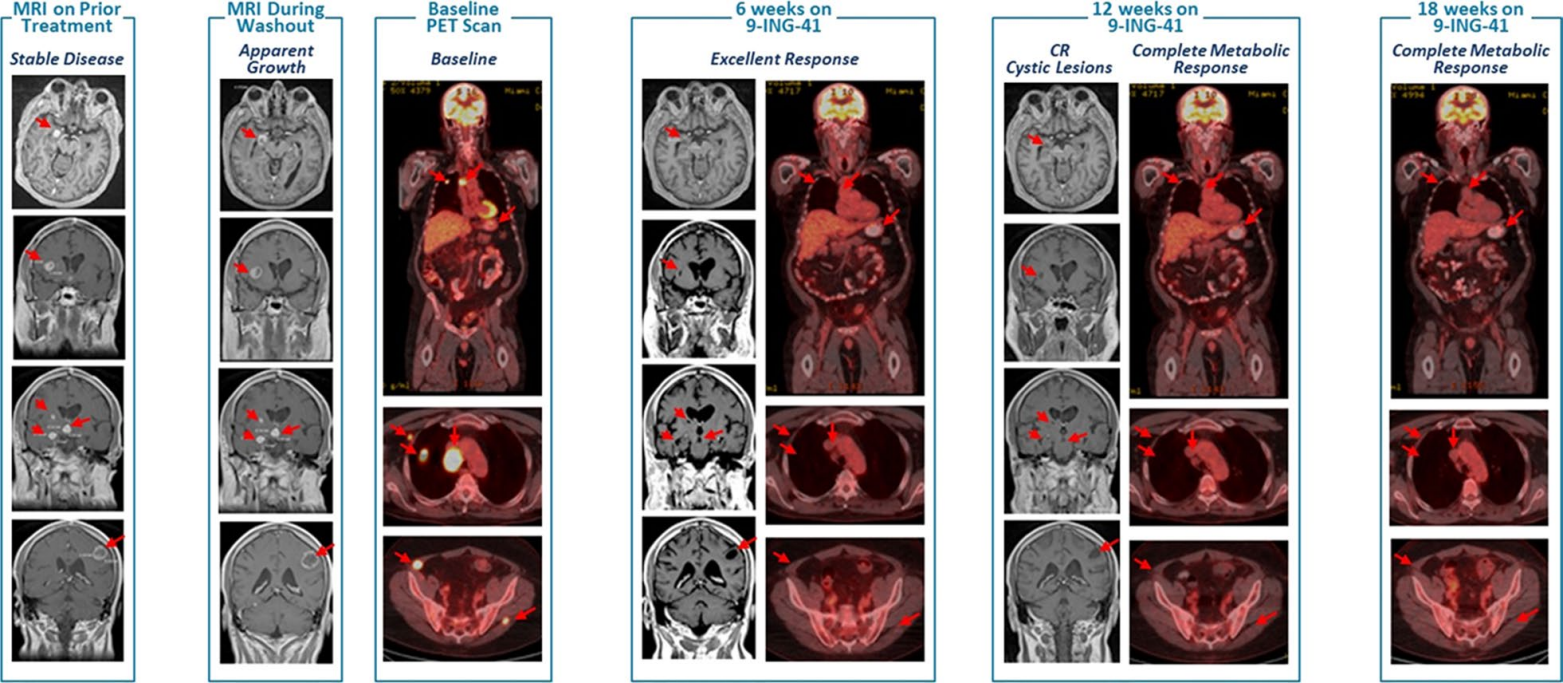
Clinical response to elraglusib monotherapy in a patient with refractory melanoma. 54-year-old male with BRAFV600E refractory melanoma and multiple sites of disease, including multiple brain lesions and progressing on anti PD-1 plus BRAF/MEK inhibitor combination. After 4-week washout brain lesions progression evident on MRI. The patient was treated with single-agent elraglusib at 5 mg/kg and within 6 weeks most sites of disease had resolved by PET, as well as significant CNS tumour shrinkage. Post-12 weeks of therapy, there was complete resolution of lesions by PET and only cystic lesions noted on brain MRI. The patient continues in CR for over 36 months
